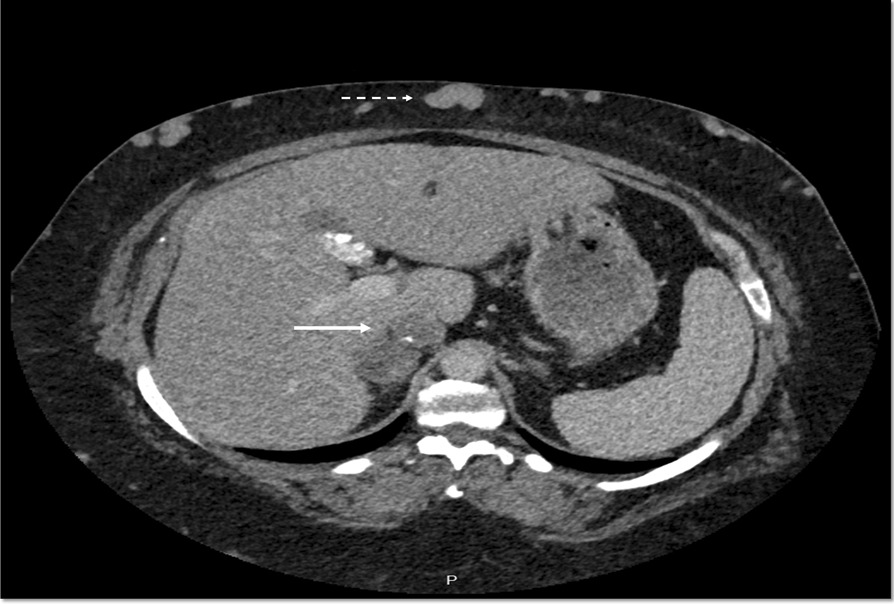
Contrast-enhanced CT scan of patient 1: IVC thrombosis (bottom arrow) and collateral abdominal veins (top, notched arrow) are clearly visible
